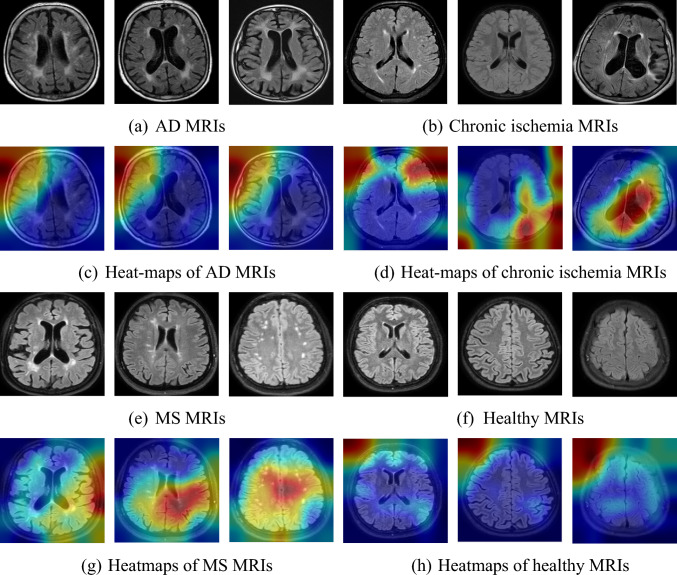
Grad-CAM results obtained for sample MRI images
